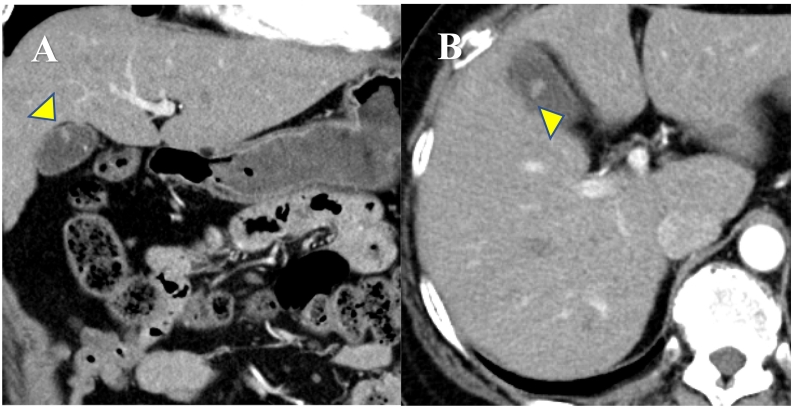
Abdominal computed tomography findings. A B: The arterial phase showing a slightly enhanced low-density tumor, 8 mm in size, located at the bottom of the gallbladder.
